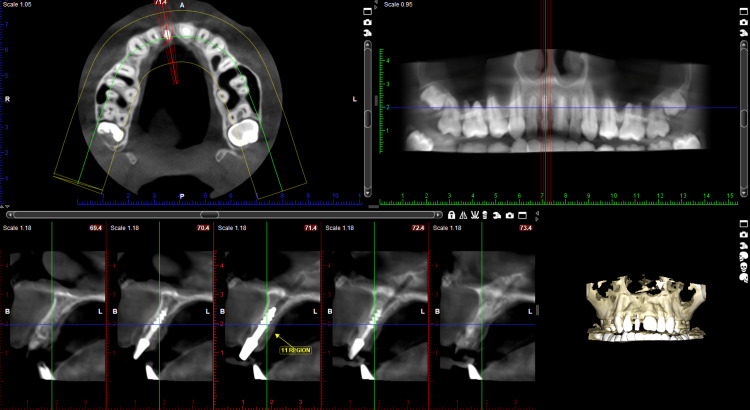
Postoperative CBCT showing the basal implant in the 11th region CBCT: Cone beam computed tomography Buccal and lingual cortical engagement seen in CBCT with basal implant in the 11th region
